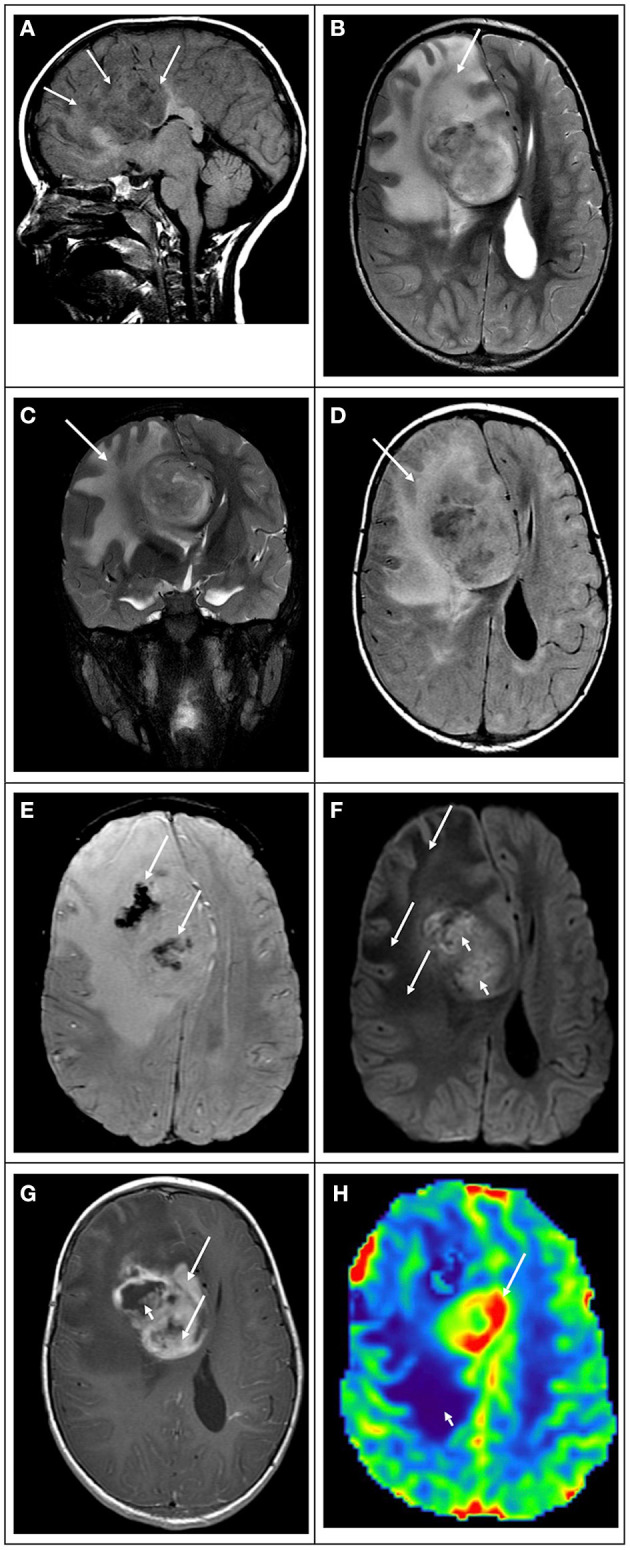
5-year-old male with headaches and confusion due to a right frontal “glioblastoma.” (A) T1 weighted image in the sagittal plane shows that the lesion is heterogeneous, ill-defined with mixed-signal, predominantly hypointense, and infiltrates the corpus callosum (white arrows). (B) T2 weighted image in the axial plane, (C) T2 weighted image in the coronal plane, and (D) FLAIR image in the axial plane show that the bulk of the lesion is hyperintense, with marked mass effect and vasogenic edema (white arrows). (E) Susceptibility weighted image in the axial plane shows irregular areas of signal drop within the tumoral bed (white arrows) in keeping with hemorrhage. (F) Diffusion-weighted image in the axial plane shows that the lesion is heterogeneous in signal. The extensive area of vasogenic edema shows low signals (white arrows). The midline component shows areas of hyperintense signal with low values in the ADC map (not shown) in keeping with restricted diffusion. (G) T1-weighted contrast-enhanced image in the axial plane shows that the tumor enhances heterogeneously. The midline component of the mass shows thick and irregular enhancement (white arrows) with no foci of enhancement in areas of necrosis (white arrowhead). (H) Arterial spin labeling perfusion image in the axial plane shows that the midline component has marked increased perfusion (white arrow). Note that the area of vasogenic edema is not associated with increased perfusion (white arrowhead).
